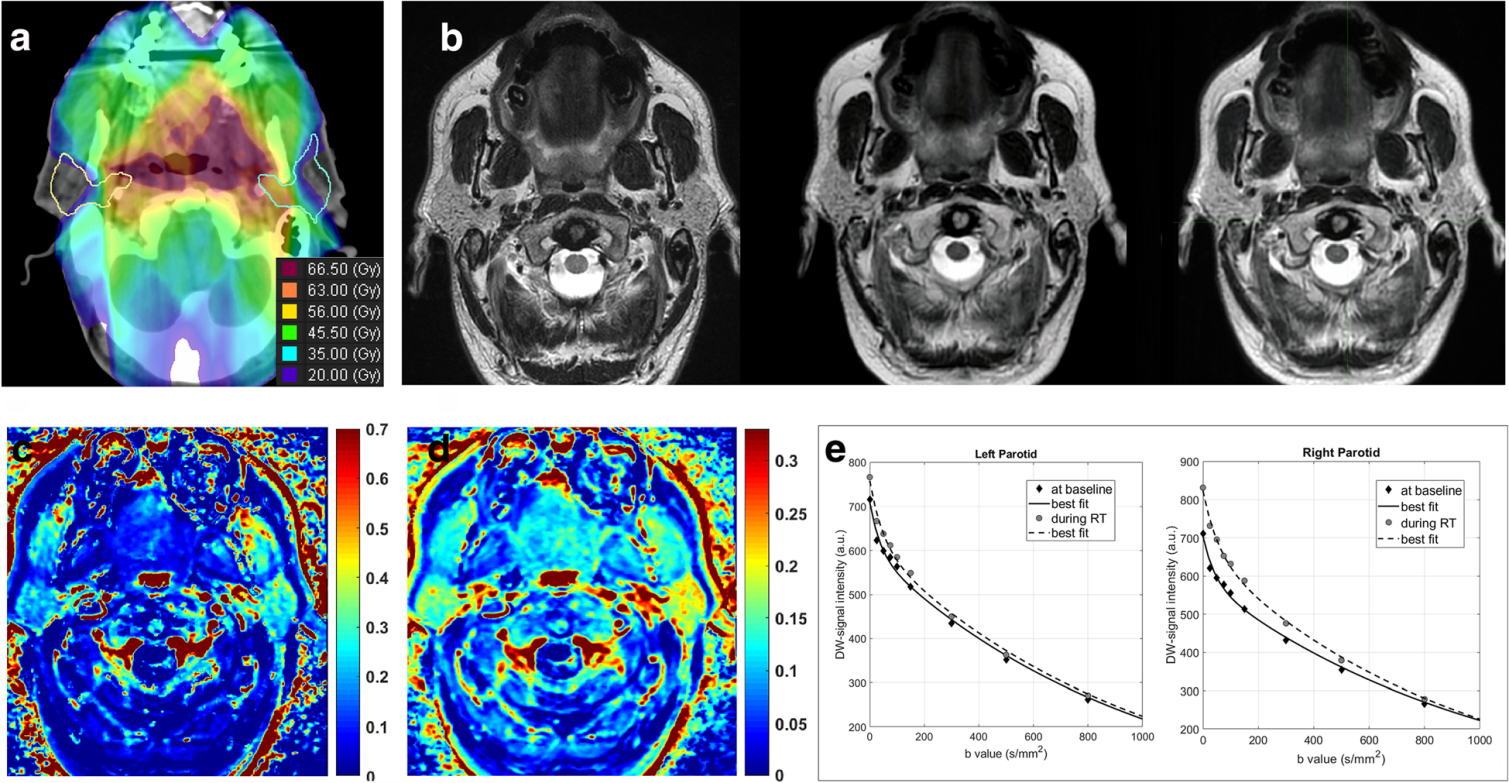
A 48-year-old man affected by a base of the tongue carcinoma: dose distribution overlaid on the central section of the parotid glands on axial CT (a); T2-weighted images before, at 2 weeks of radiotherapy and 8 weeks after radiotherapy (b) showing a small final parotid shrinkage; the corresponding maps of K^trans^ in min^− 1^ (c) and IAUGC in a.u. (d), at baseline, indicating moderately perfused glands. Correspondingly, a slight difference was observed between the rates at which the DW-signal attenuation of the parotids decreases during RT (e)
